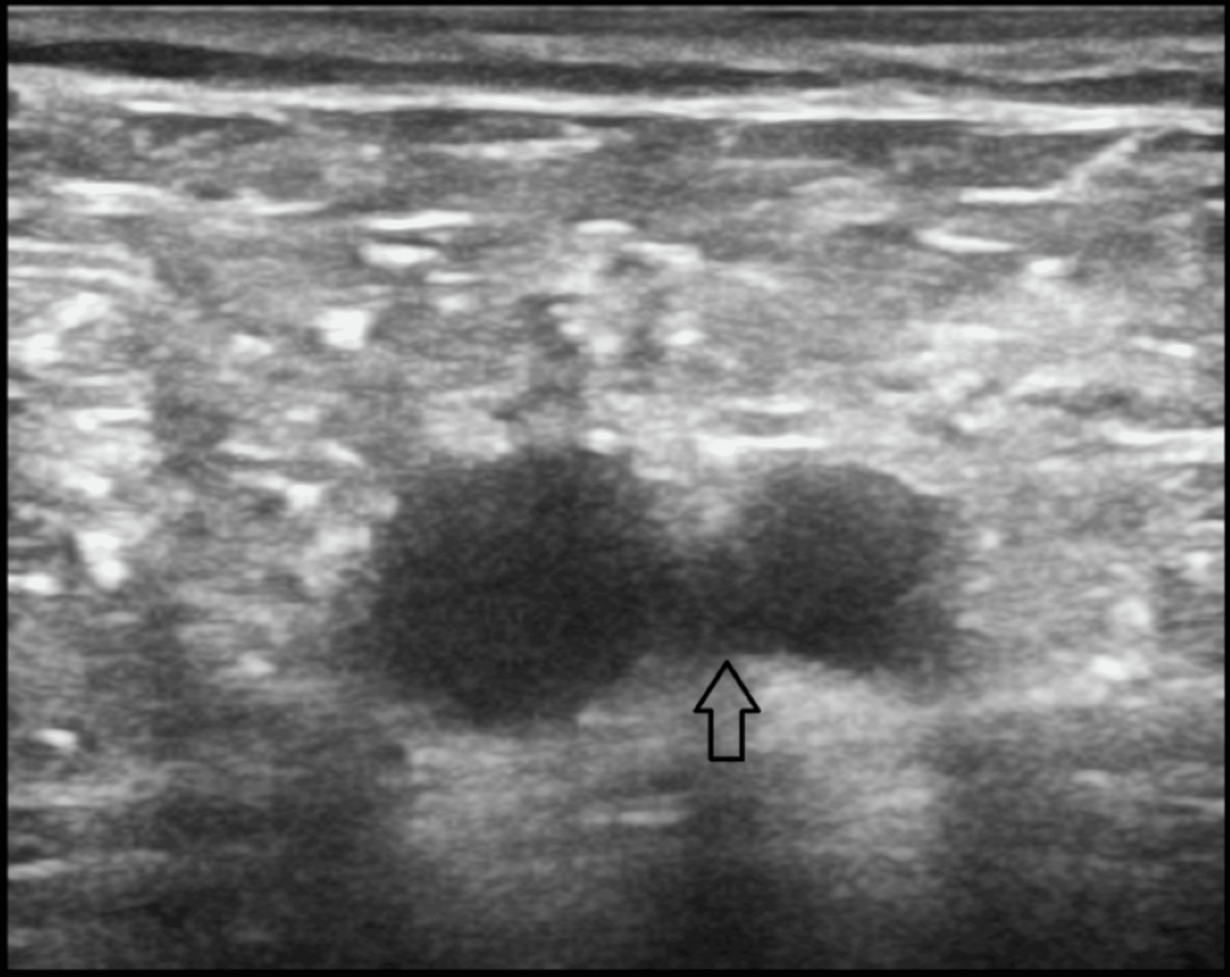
Lower limb venous Doppler studies revealed no thrombus - negative deep venous thrombosis
